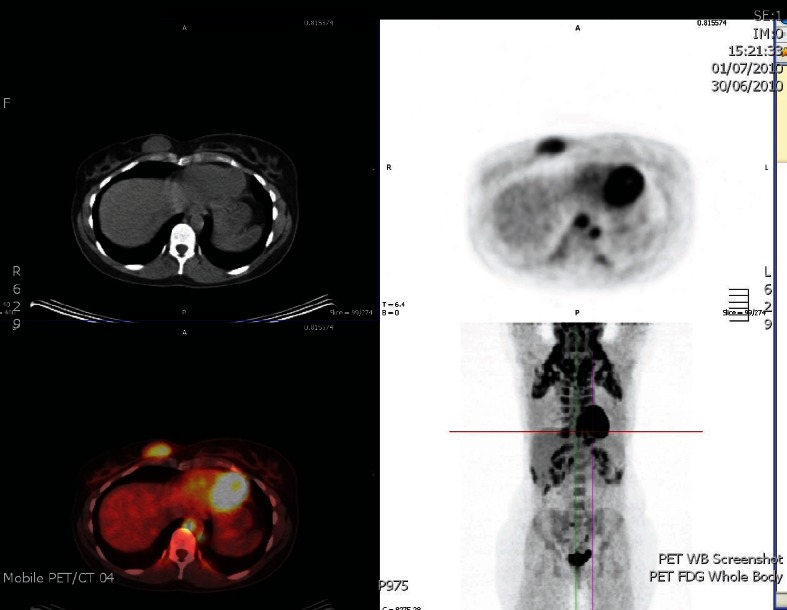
MRI scan of right breast shows well circumscribed solid mass.
